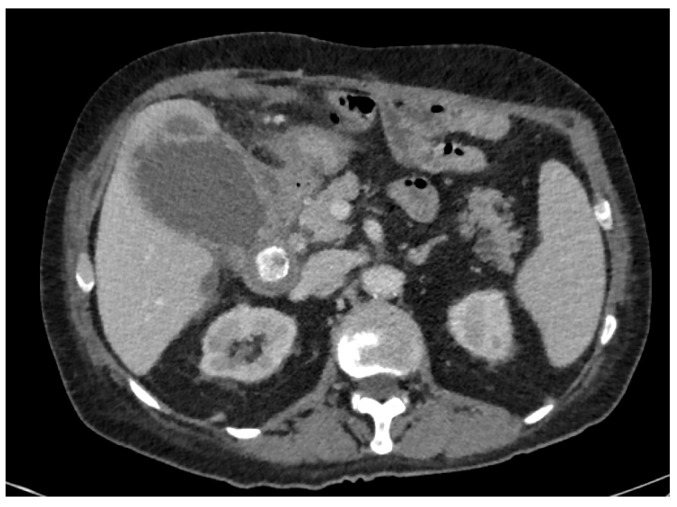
Initial CT scan in a 73-year-old man, known for ischemic and rhythmic heart disease anticoagulated with Apixaban, with an ASA score = 3, presenting pain for 2 weeks, with a significant inflammatory syndrome (CRP = 251 mg/L).
